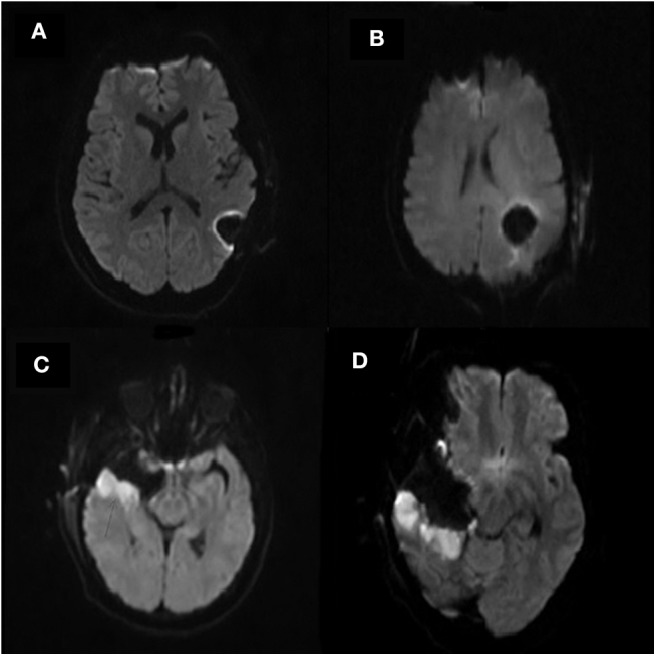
(A–D) Selected example post-operative diffusion (b1000) MRI scans of four separate subjects. (A,B) are representative of subjects in the control cohort. Note the resection cavities seen in the left parietal lobe on both scans which exhibit a minimal rim of hyperintensity surrounding the resection cavity. (C,D) are representative of subjects in the infarct cohort. Note the resection cavity seen in the right temporal lobe on both scans. Also note the larger area of diffusion hyperintensity surrounding the resection cavity representing arterial infarcts.
